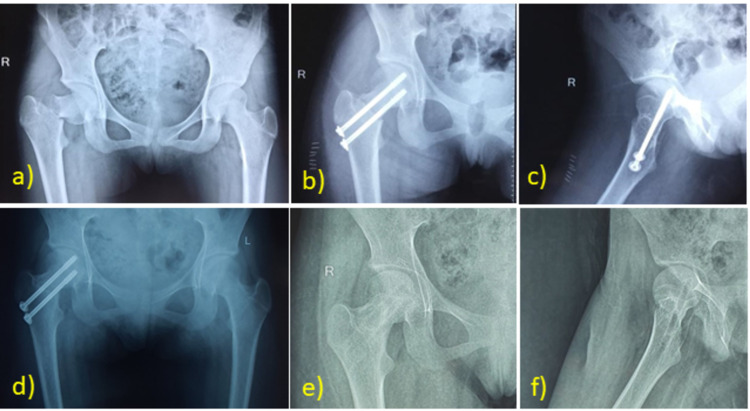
Case Example: Closed Reduction and Fixation With Screws A) AP radiograph of a 15-year-old female patient with Delbet type III fracture presented 14 hours after the injury and was taken immediately to the operating room for fixation. Postoperative AP (b) and lateral (c) radiographs of the proximal femur after closed reduction and internal fixation with cannulated screws through an anterolateral approach. d) AP radiograph showing union at three months follow-up. e) AP and (f) lateral radiographs demonstrating no signs of osteonecrosis after implant removal at one year. AP: anteroposterior
